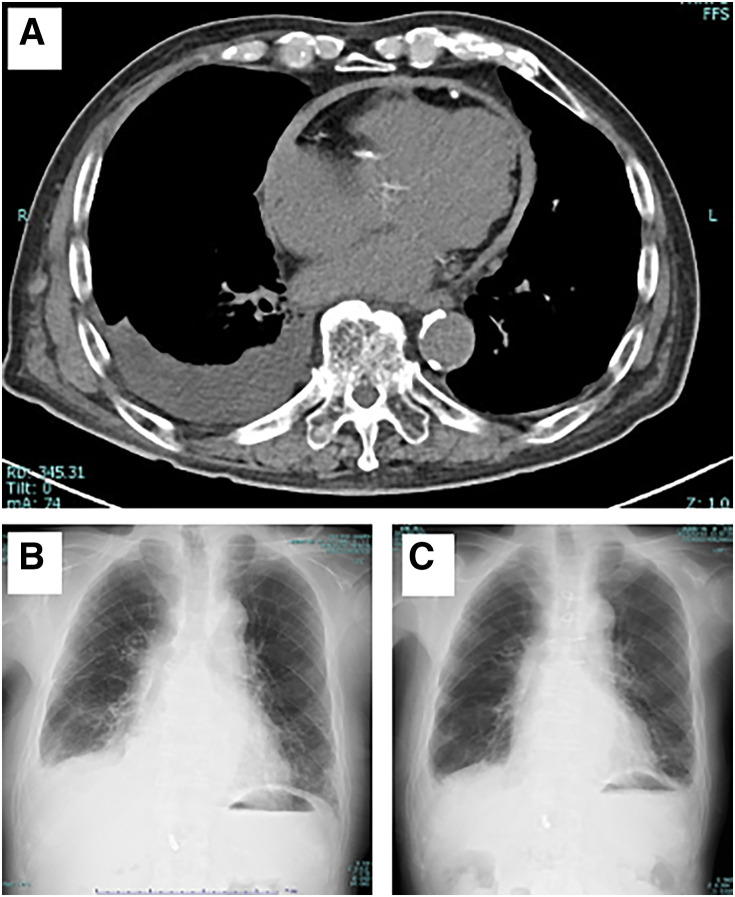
Preoperative imaging findings. (A) Preoperative CT image showing diffuse pericardial thickening. (B) Preoperative chest radiograph demonstrating cardiomegaly and clear lung fields. (C) Postoperative chest radiograph showing a reduced cardiac silhouette and no evidence of diaphragmatic elevation, suggesting absence of phrenic nerve injury.
